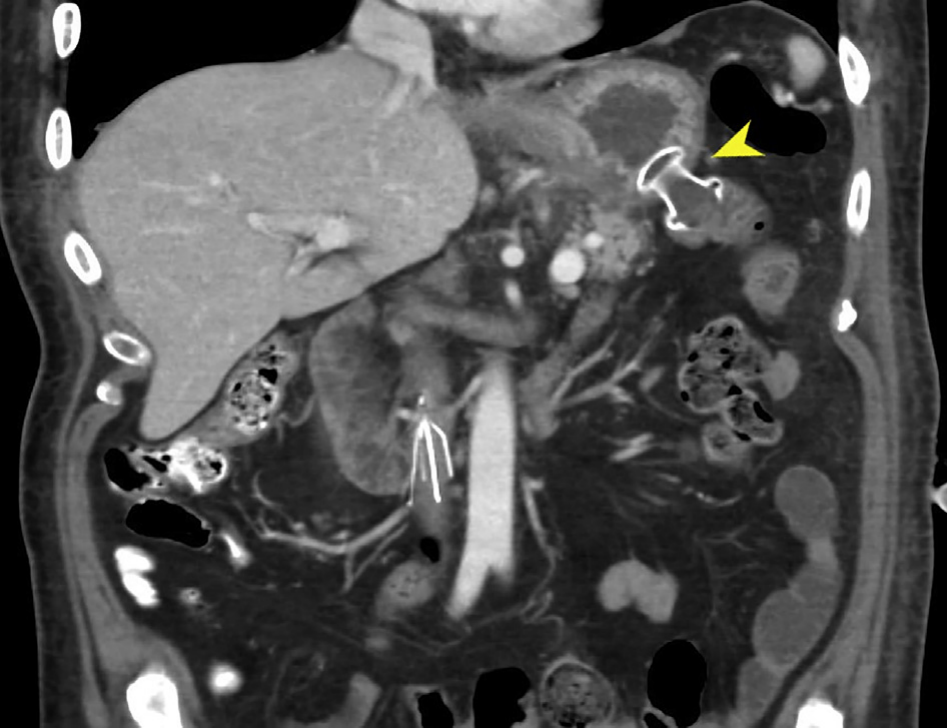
Postoperative CT image of the gastroenterostomy (*yellow arrowhead*).
